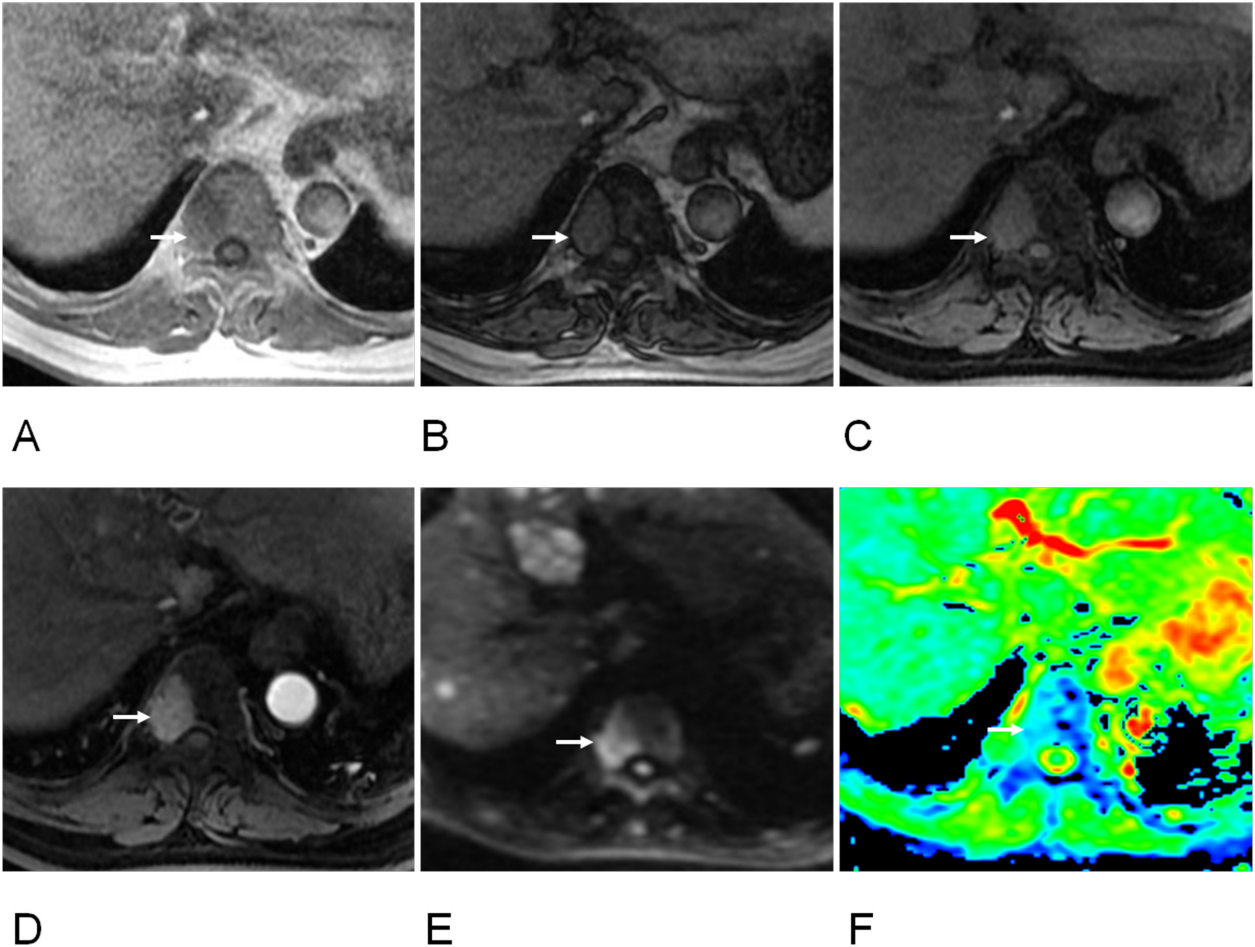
63-year old women of hepatocellular cell carcinoma with metastasis of T9 vertebra (A) T1-weighted image showed a hypointense signal lesion on T9 vertebra (arrow). (B) Out-phase image showed hyperintense signal (arrow), ratio of SI loss between in- and out-phase was 0.01. (C) FS T1-weighted images showed hyperintense signal (arrow), ratio of SI loss between T1 and FS T1 was 0.16. (D) Early enhanced image showed hyperintensity (arrow) (enhancement ratio, 0.90; SIR, 4.26). (E) DW image showed hyperintensity at b value of 1500 s/mm^2^ (arrow). (F) Apparent diffuse coefficient (ADC) was 0.61 x10^-3^ mm^2^/s (arrow).
